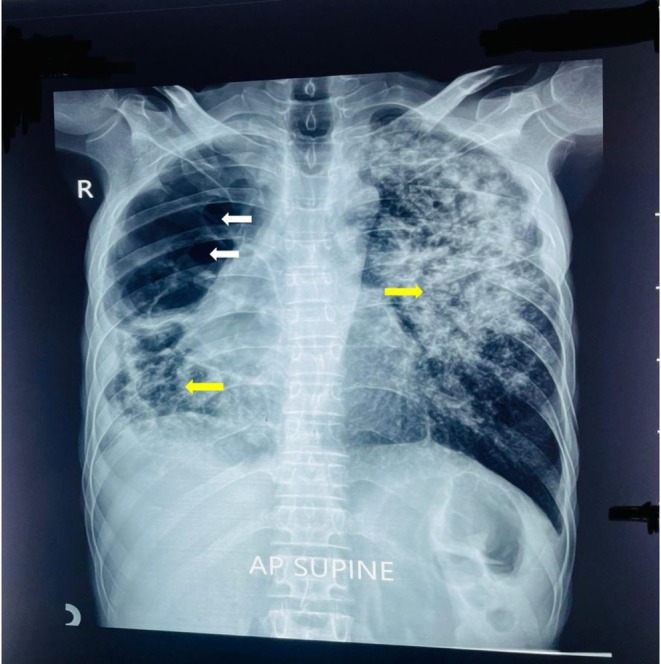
Chest radiograph* (*anteroposterior view*) *demonstrating extensive bilateral structural lung disease*. Heterogeneous opacities are seen predominantly in the left upper lung zone, with additional patchy opacities in the right mid and lower zones (yellow arrows). Multiple rounded lucent areas in the right upper lung zone suggestive of bullous changes (white arrows).
